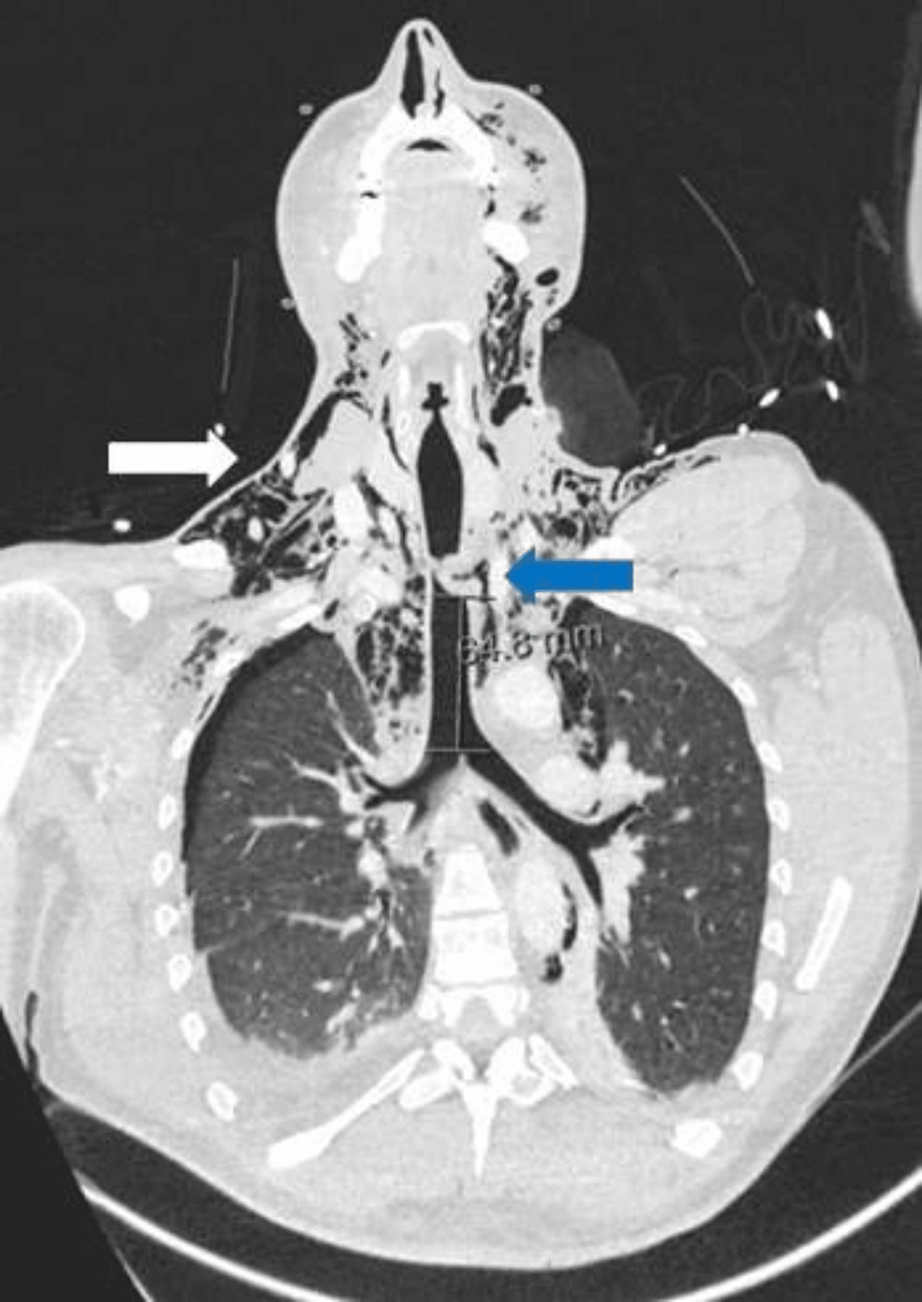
Admission thoracic CT: Left anterolateral tracheal laceration in the thoracic portion (blue arrow); subcutaneous emphysema (white arrow)
